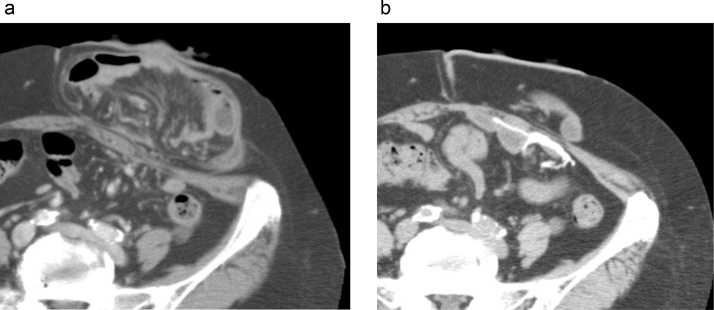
CT images of case 2. a. Preoperative abdominal CT demonstrated large parastomal hernia with herniation of the small intestine into the subcutaneous space. b: CT image 1 year after the repair demonstrated no recurrence of parastomal hernia.
